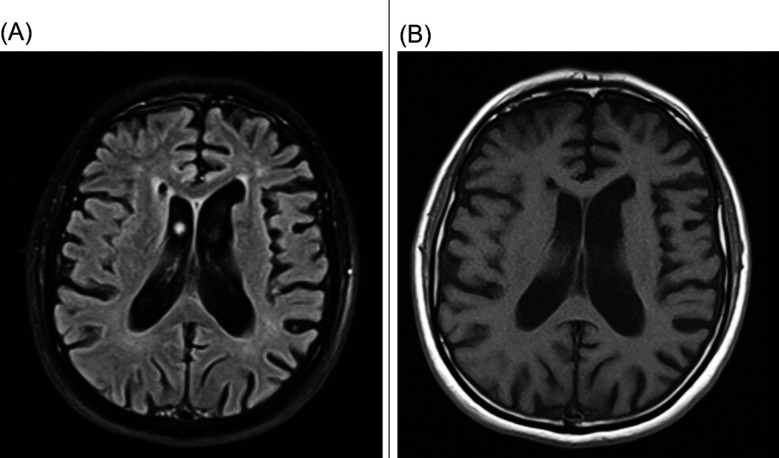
Brain magnetic resonance imaging revealing bilateral temporal lobe atrophy. A) Fluid attenuated inversion recovery. B) T1-weighted axial sections.
